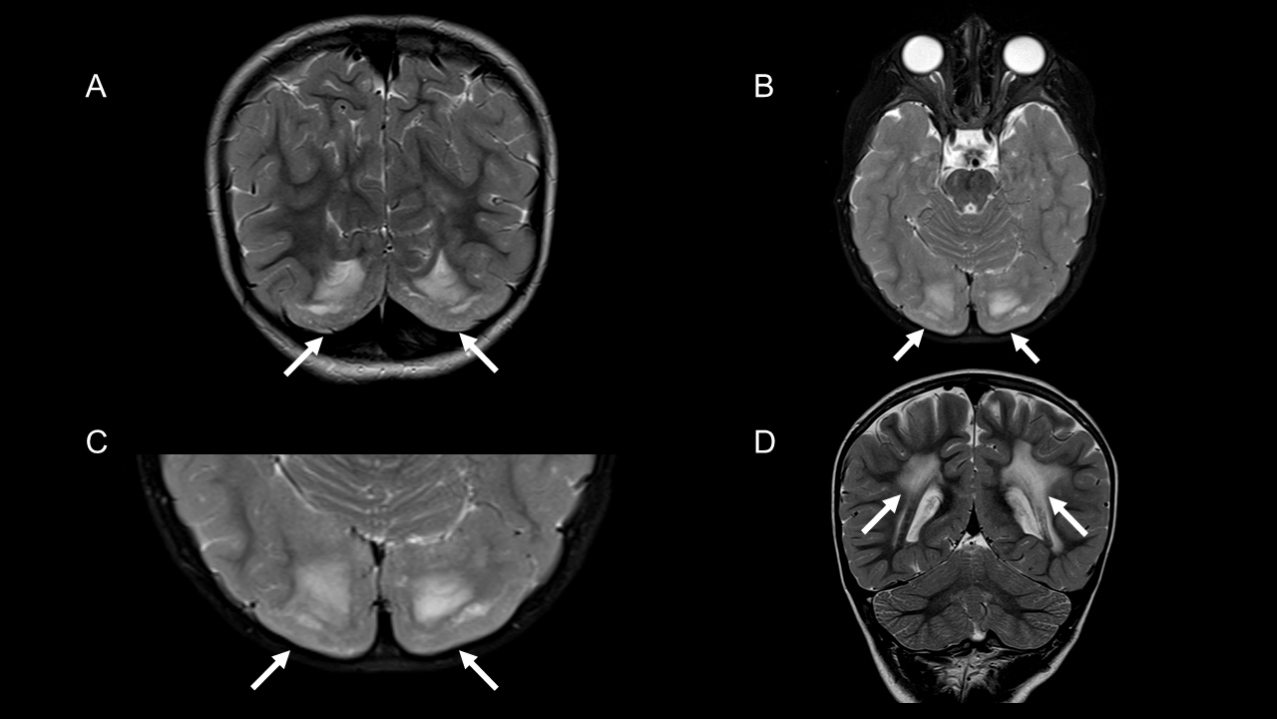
(A) Coronal T2-weighted image (T2WI) showing bilateral and symmetric type II lissencephaly or “cobblestone” lissencephaly (arrows). (B) Axial T2-weighted image (T2WI) showing bilateral and symmetric type II lissencephaly or “cobblestone” lissencephaly (arrows). (C) Axial T2-weighted image (T2WI) with a closer view of the symmetric type II lissencephaly or “cobblestone” lissencephaly (arrows), in contrast with normal cortical development. (D) Coronal T2-weighted image (T2WI) showing signal abnormalities in the periventricular white matter (arrows).
